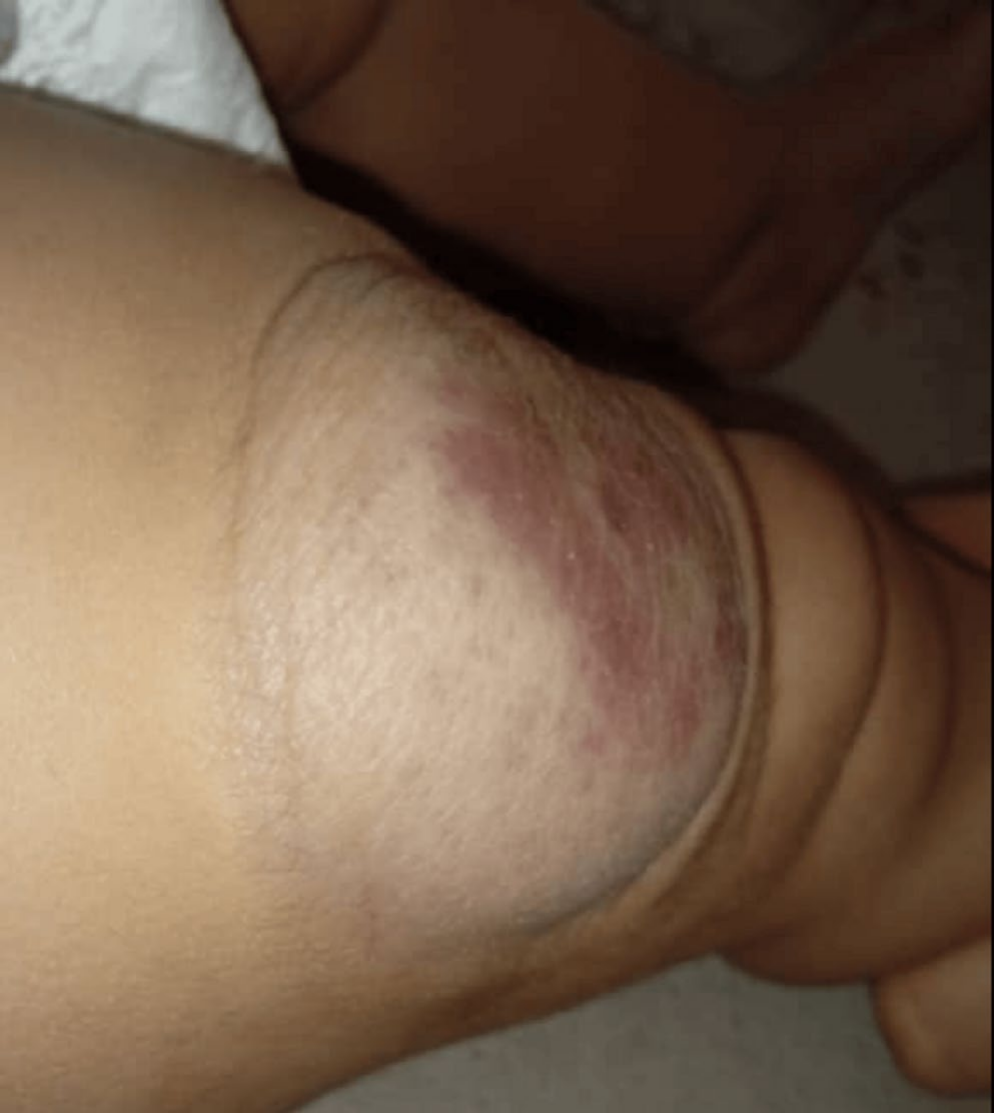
Post‐treatment status of the hemangioma following ultrasound‐guided percutaneous sclerotherapy with bleomycin. The image shows a significant reduction in the size of the lesion, with improved skin texture and color.
